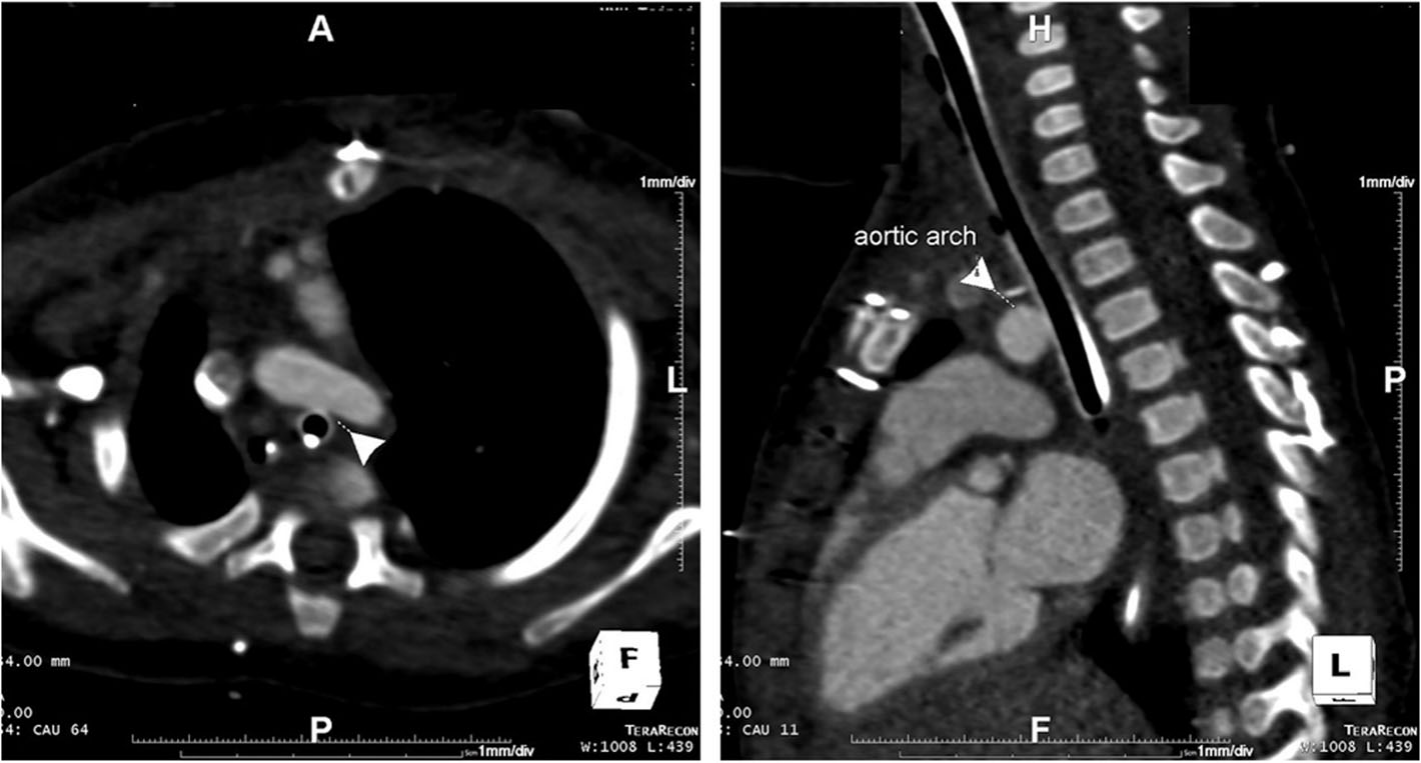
Contrast-enhanced computed tomography angiography (CTA) showed poor differentiation of the borders between posterior wall of distal aortic arch and anterior wall of lower trachea, but with no contrast extravasation into airway (white arrow indicates aortic arch)
